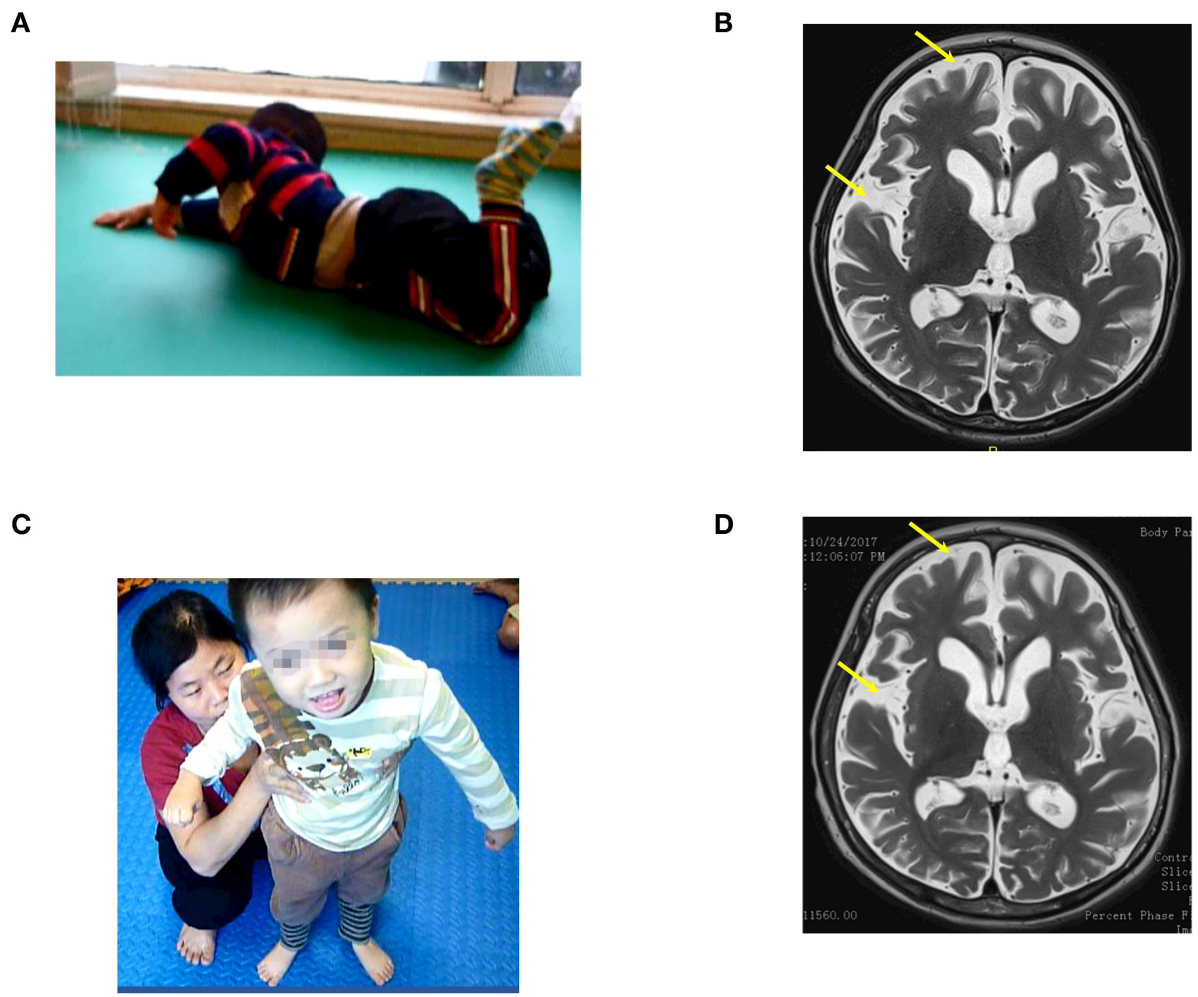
The patient (case 4) before and after autologous BMMNC transplantation. (A) Nine months after the drowning before BMMNC transplantation, the patient had no reaction during communication and could not follow objects and speak and exhibited muscle spasticity at level V. He could not sit up or crawl and had epilepsy. (B) Brain MRI revealed diffuse cerebral atrophy before transplantation (arrows). (C) Six months after the third transplantation, the patient's cognition improved progressively and could stand with support. (D) However, brain MRI showed no significant change after transplantations.
